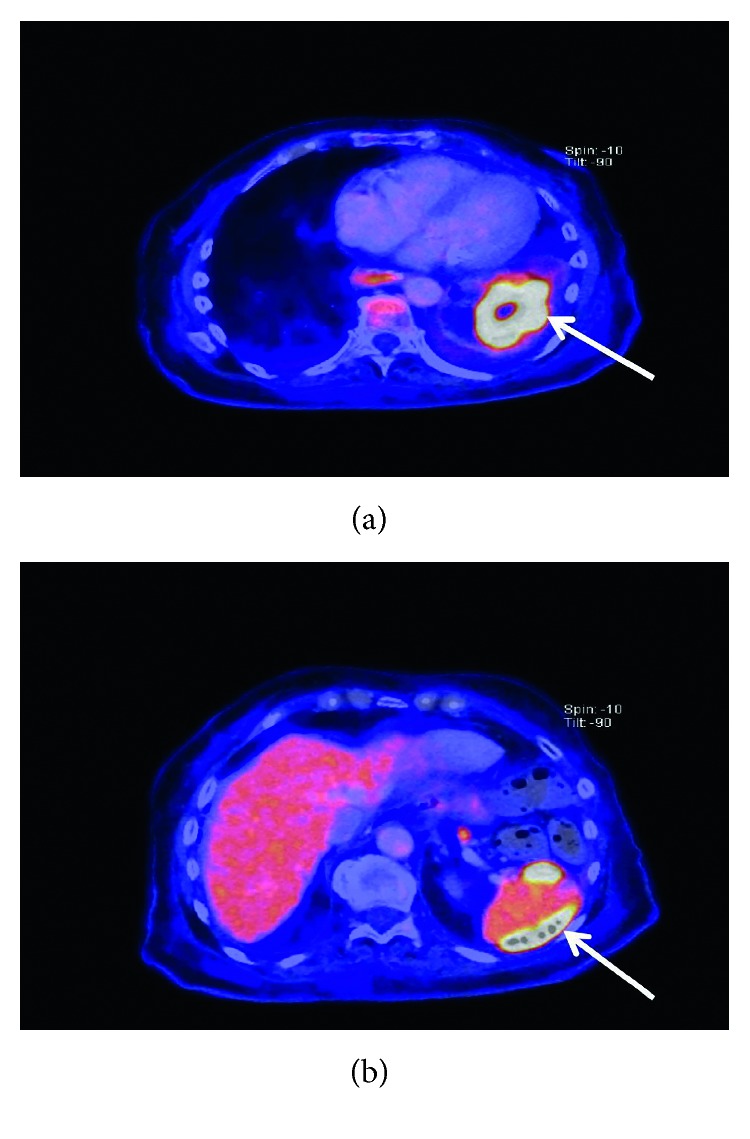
(a) PET/CT scan showing slightly increased 18F FDG uptake of the spleen performed during the antibiotic therapy indicates inflammation. (b) PET/CT scan showing splenic inflammation as well as splenic abscesses.
